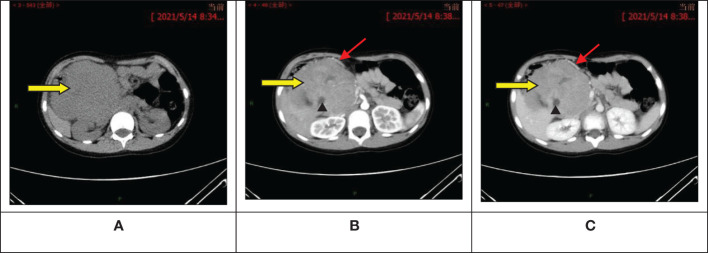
Abdominal computed tomography scan + enhancement show a primarily solid, mixed-density mass in the pancreatic head area, with significant enhancement of the solid component, interspersed low-attenuation foci, and visible tortuous small vessels within. (A) Pre-contrast phase; (B) Artery stage; (C) Portal vein stage.
